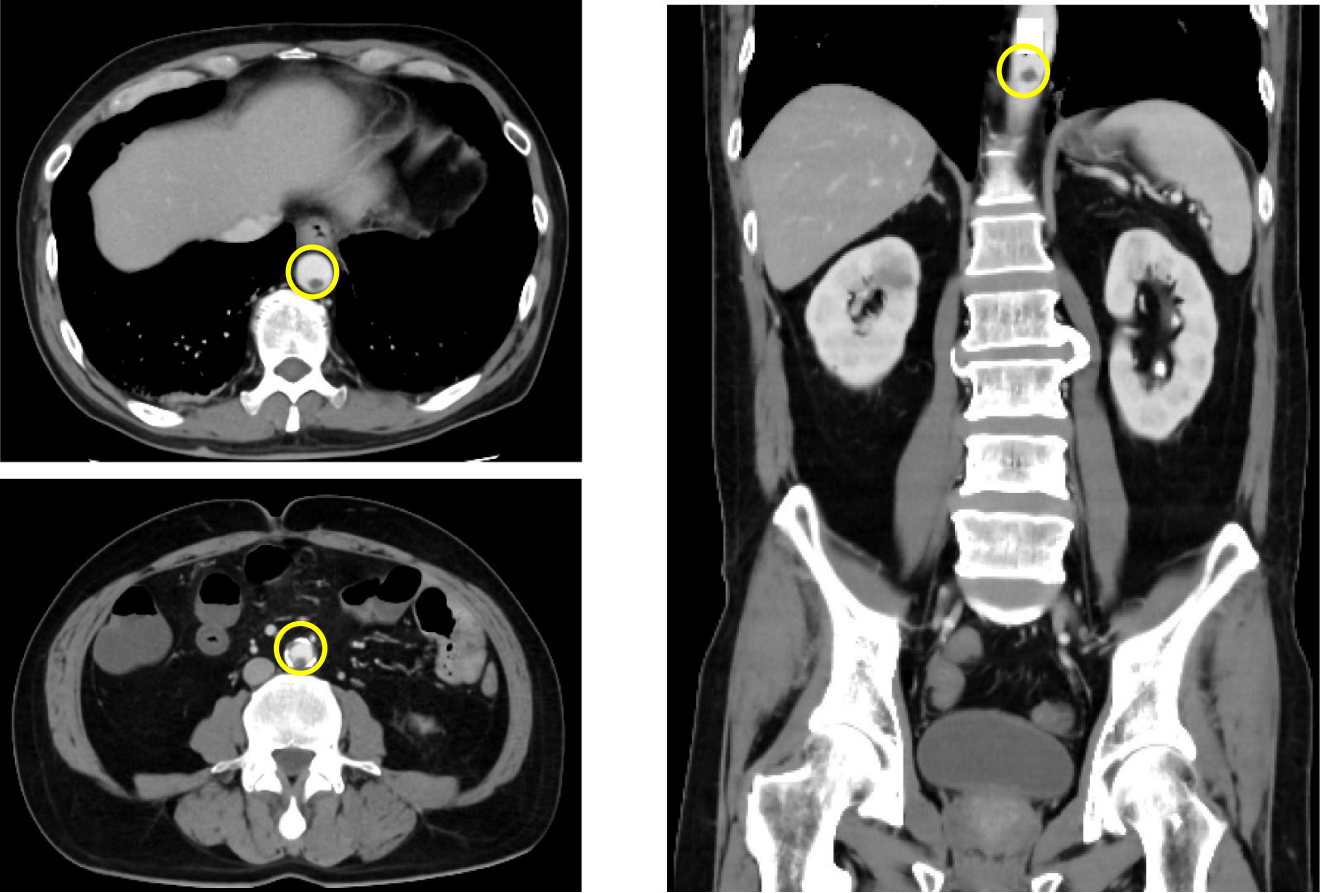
Abdominal contrast-enhanced CT findings (6th day after hospitalization): Multiple thrombosis from the descending aorta to the abdominal aorta (○). No obvious evidence of intestinal ischemia was observed
